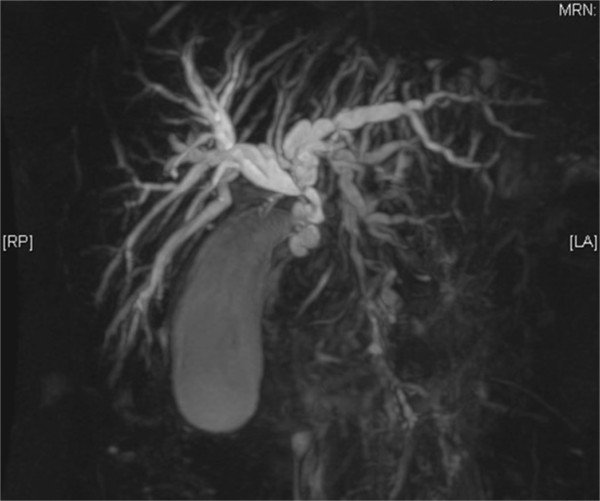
Magnetic resonance imaging showing the stenosis of the middle portion of the common bile duct and the dilatation of intra- and extra-hepatic biliary tree.
